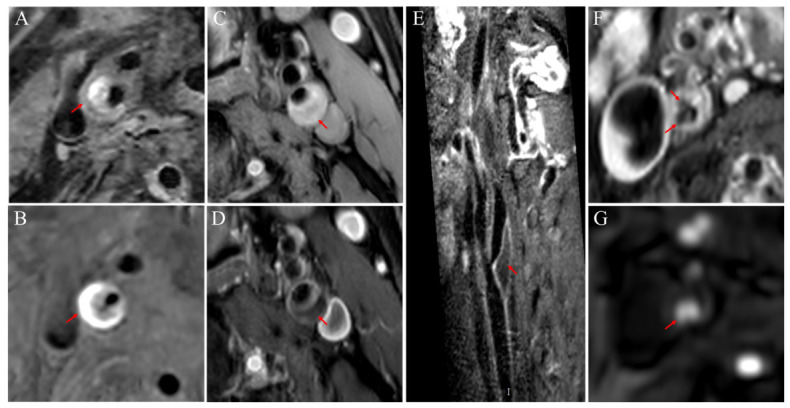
Representative HR-MRI findings of carotid plaque vulnerability. (A,B) Axial non-contrast T1-weighted image and simultaneous non-contrast angiography and intraplaque hemorrhage (SNAP) image showing intraplaque hemorrhage (IPH). (C–E) Axial non-contrast T1-weighted image, axial contrast-enhanced T1-weighted image, and sagittal contrast-enhanced T1-weighted image showing lipid-rich necrotic core (LRNC). (F,G) Contrast-enhanced T1-weighted image and time-of-flight (TOF) MR angiography showing plaque ulceration. Red arrows indicate representative plaque vulnerability features.
